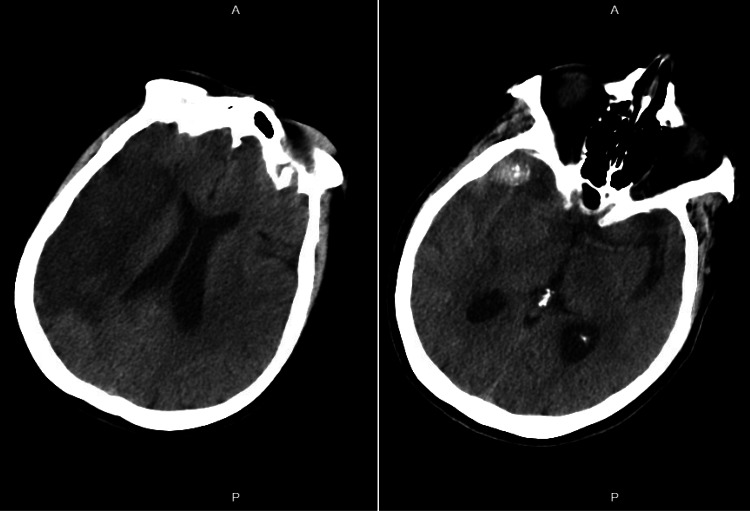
Axial CT demonstrating a hyperdense, calcified, large thrombosed MCA bifurcation aneurysm, associated with infarction and extensive cytotoxic edema in the MCA territory MCA: middle cerebral artery
